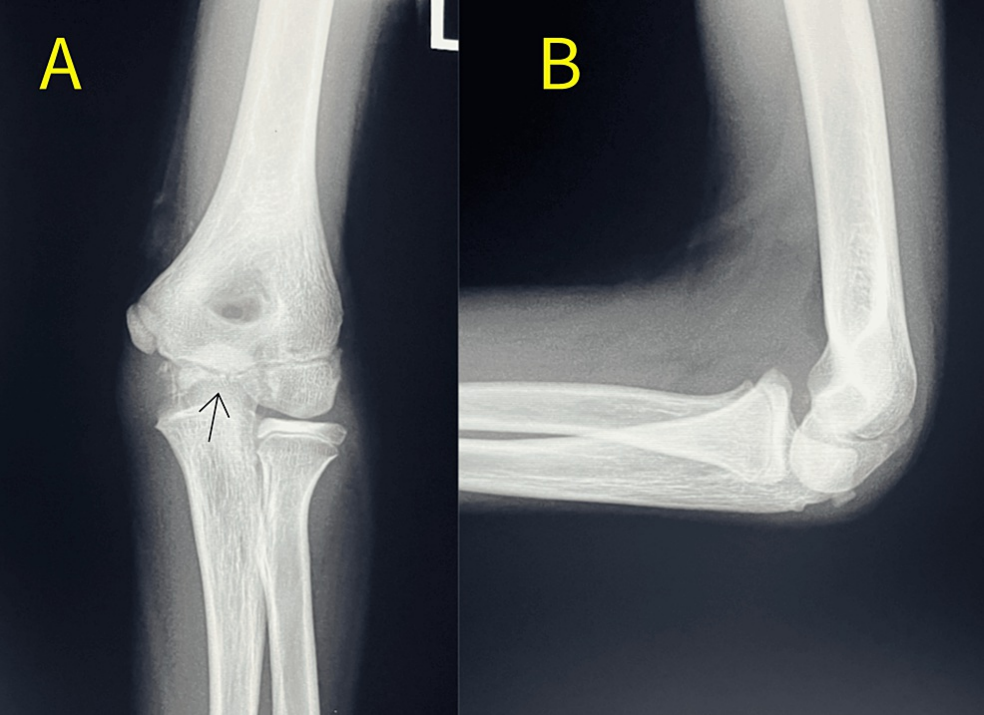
X-ray of anteroposterior (A) and lateral (B) views of the elbow joint three years after initial injury. The distal end of the humerus is not completely ossified. However, a deformity of the articular surface at the trochlea is suspected.
